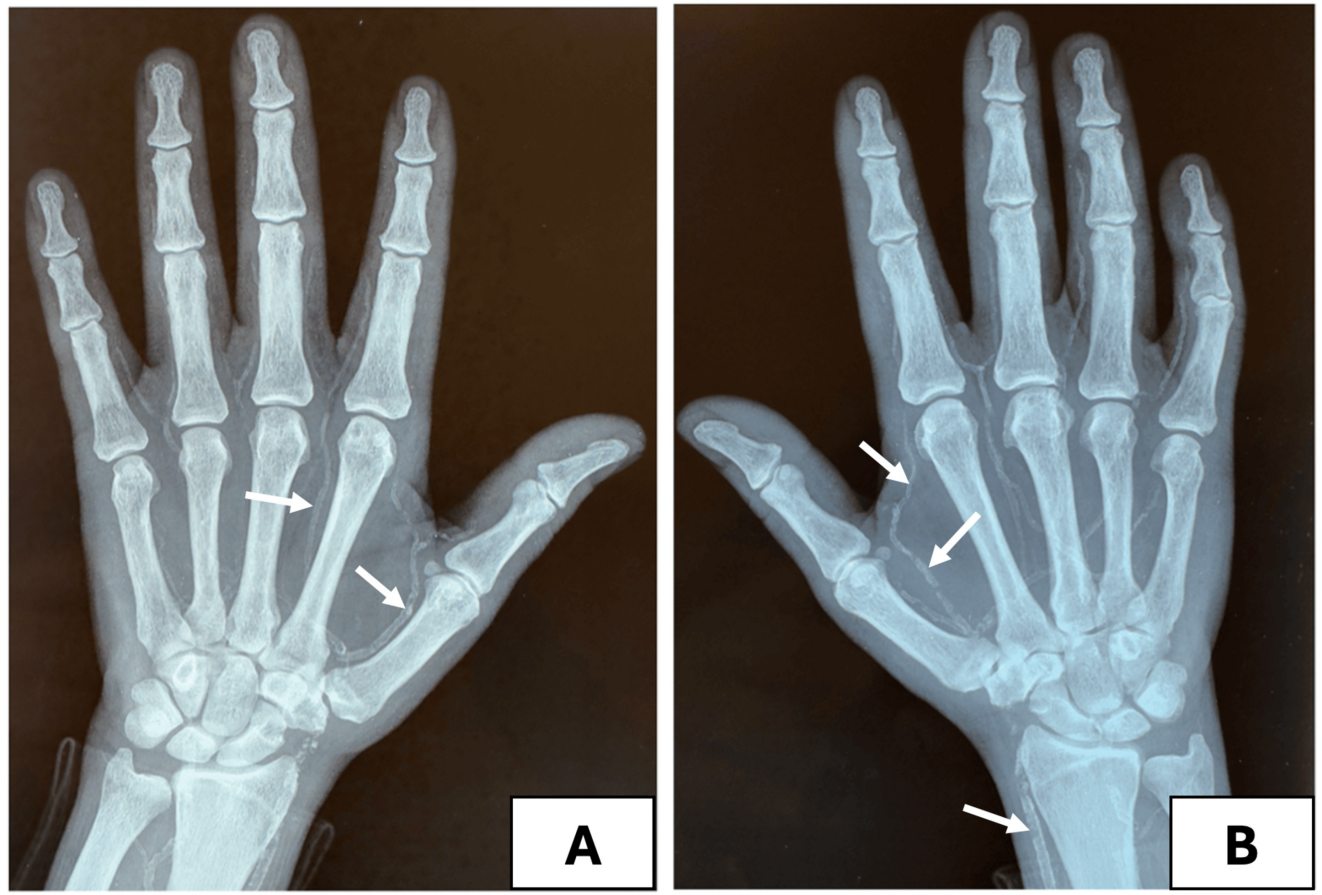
X-ray of both hands showing diffuse arterial calcifications of the upper limb with a net-like pattern observed in the hand vessels. A: Arterial calcifications of the deep palmar arch of the left hand resulting in a net-like pattern. B: Arterial calcifications of the deep palmar arch and the radial artery in the right upper limb.
